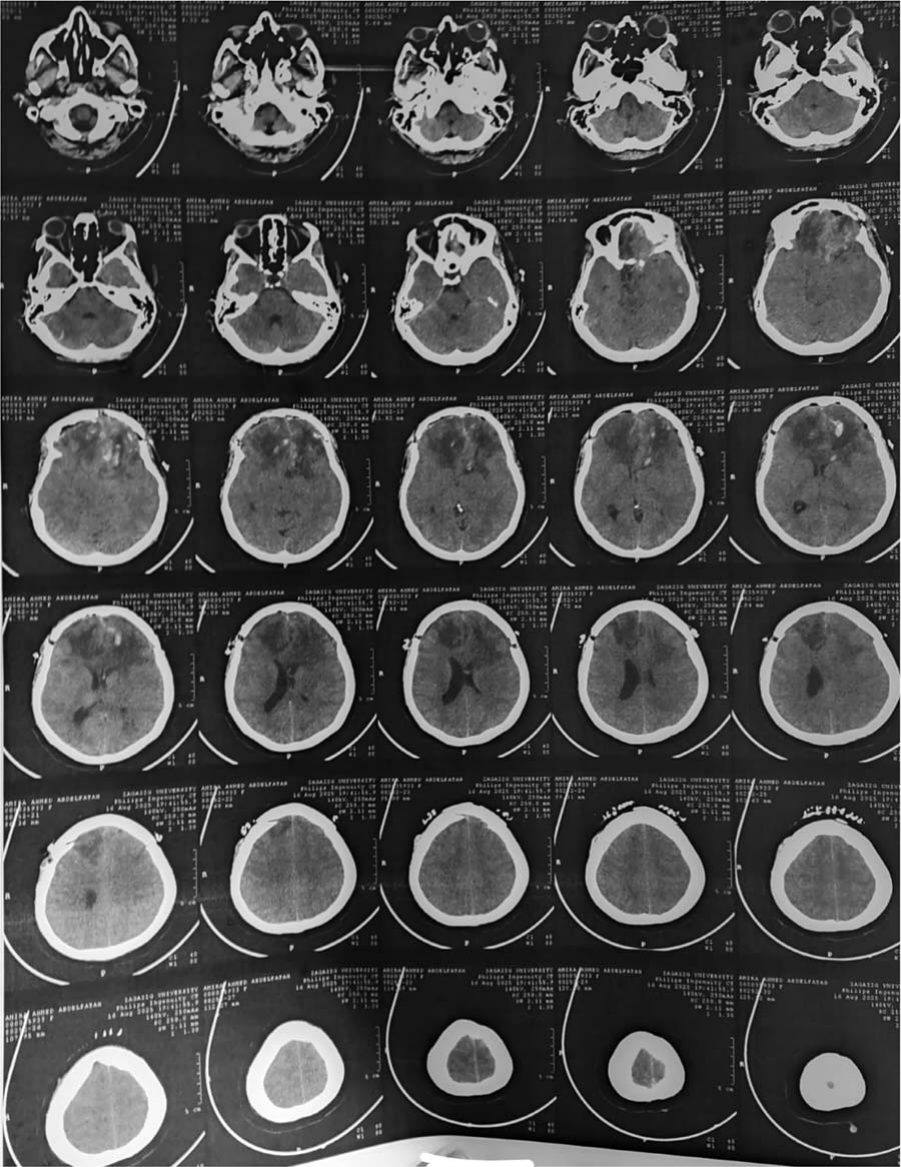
Post-op CT brain showing total excision of the tumor leaving mild post-op oedema at tumor bed.
